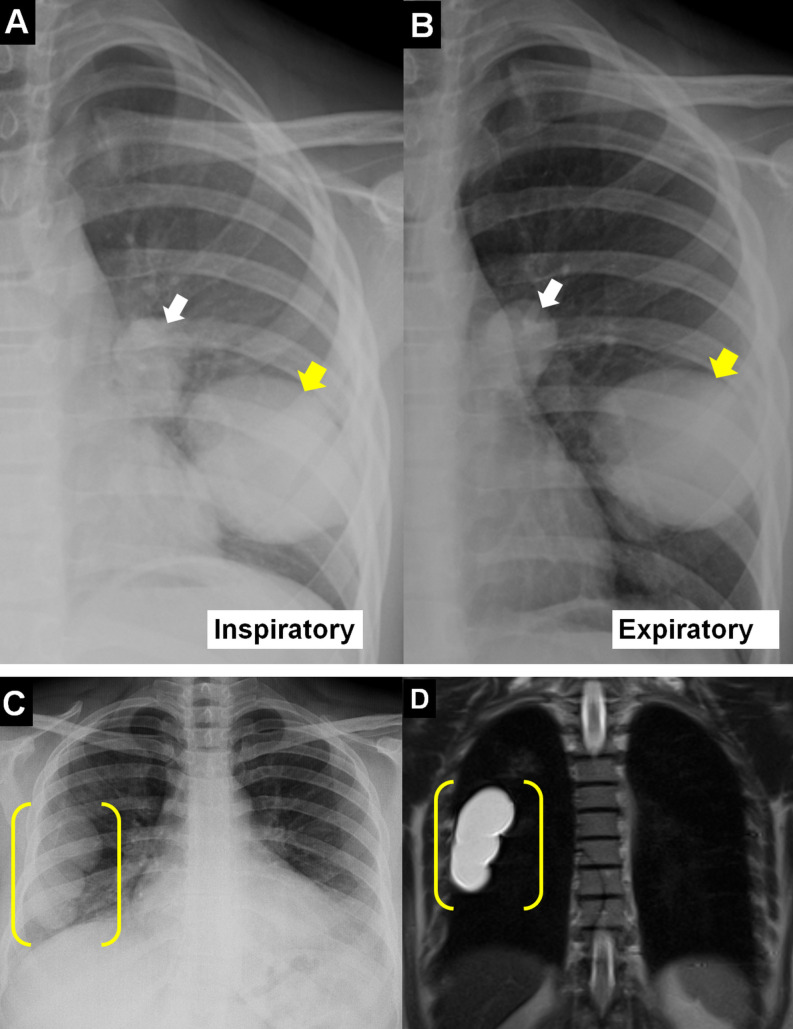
The “escudero-nemerow sign” (arrows), which becomes oval in inspiration (A) and more circular in expiration (B), is seen in intact pulmonary hydatid cysts on chest radiographs. On chest radiograph (C) and magnetic resonance imaging (D), the cyst deviates from round contours and has a conjoined lobulated appearance (frames) due to pressure on surrounding tissue or the developmental process.
